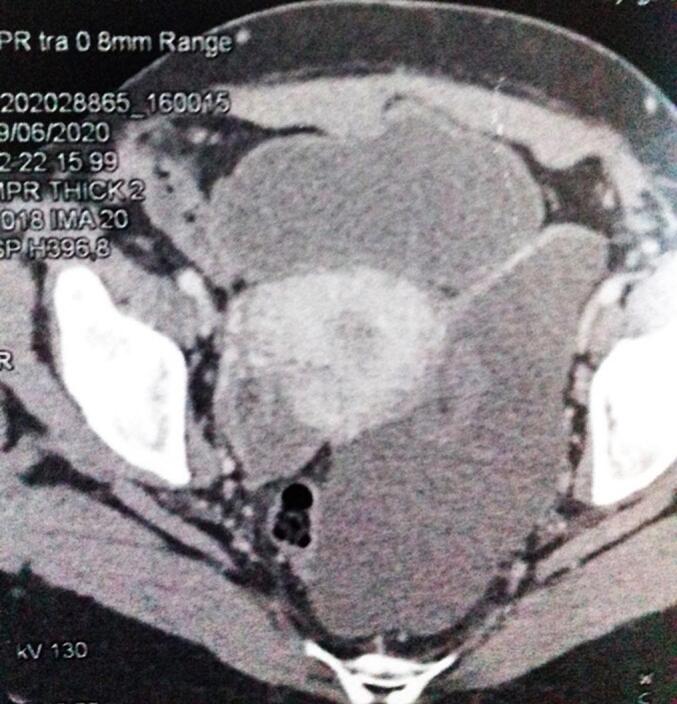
Abdominal CT scan of BMPM: polylobate hypodense formation with multiple thin internal septa which are enriched after contrast.
